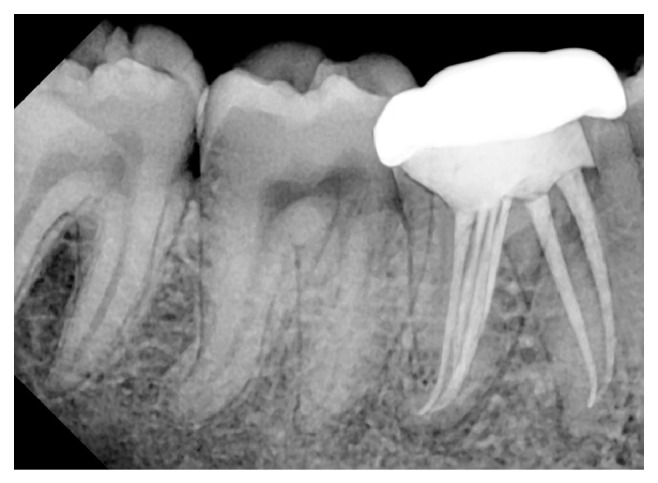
15-month follow-up radiograph.
